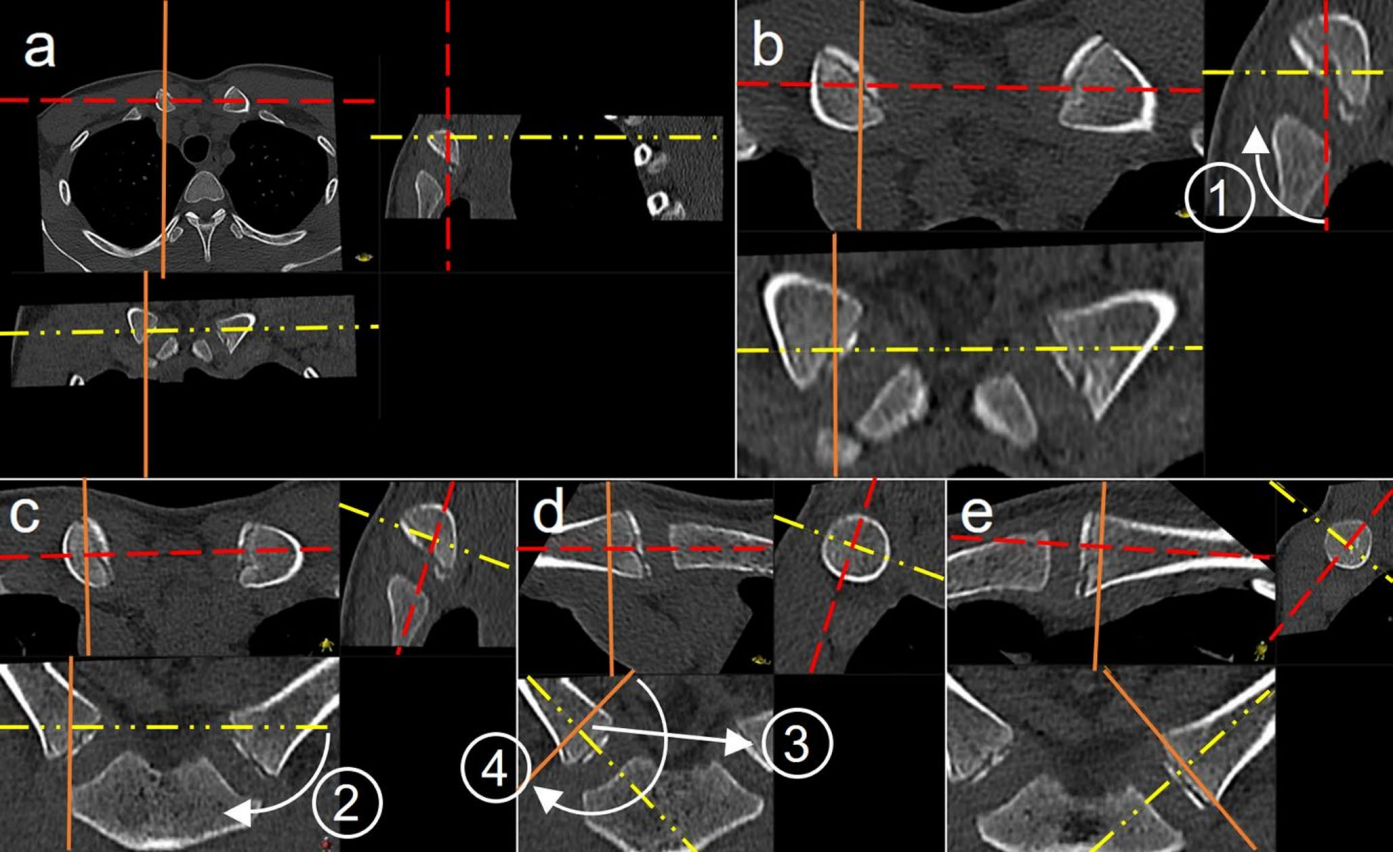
Multi-planar reconstruction of true coronal and axial views of the clavicle in MCE CT scans with arms-up. (a) Default position of CT images in the multi-planar reconstruction (MPR) viewer, with the crosshair centred on the right MCE. (b) Enlarged view of the default position. Step 1 – Adjusting to true coronal view: From the default view (b), rotate the red crosshair line clockwise in the sagittal view until it aligns with the clavicle centreline, as shown in (c). Step 2 – Generating true coronal and axial views of the right MCE: From the true coronal view in (c), rotate the yellow crosshair clockwise in the coronal view to align with the clavicle’s centreline, producing a true coronal and true axial view of the right MCE, as shown in (d). Steps 3 and 4 – Switching to true coronal and axial views of the left MCE: Move the orange and yellow crosshair lines to the left MCE. Then, rotate the orange crosshair line clockwise by 180° to achieve the orientation shown in (e)
